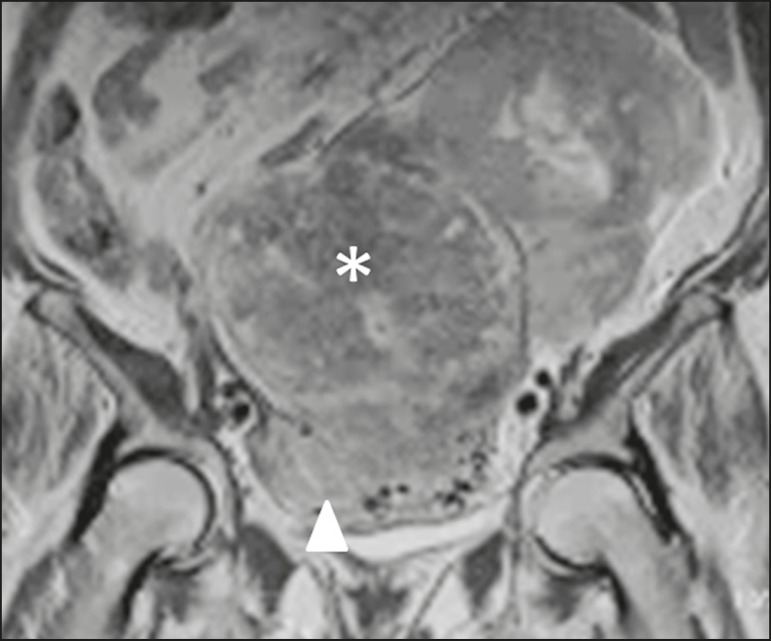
Leiomyosarcoma of the broad ligament in a 68-year-old woman. Coronal T2-weighted imaging showing a huge heterogeneous intermediate-signal solid mass in the pelvis (asterisk) with the uterus partly observed (triangle).
